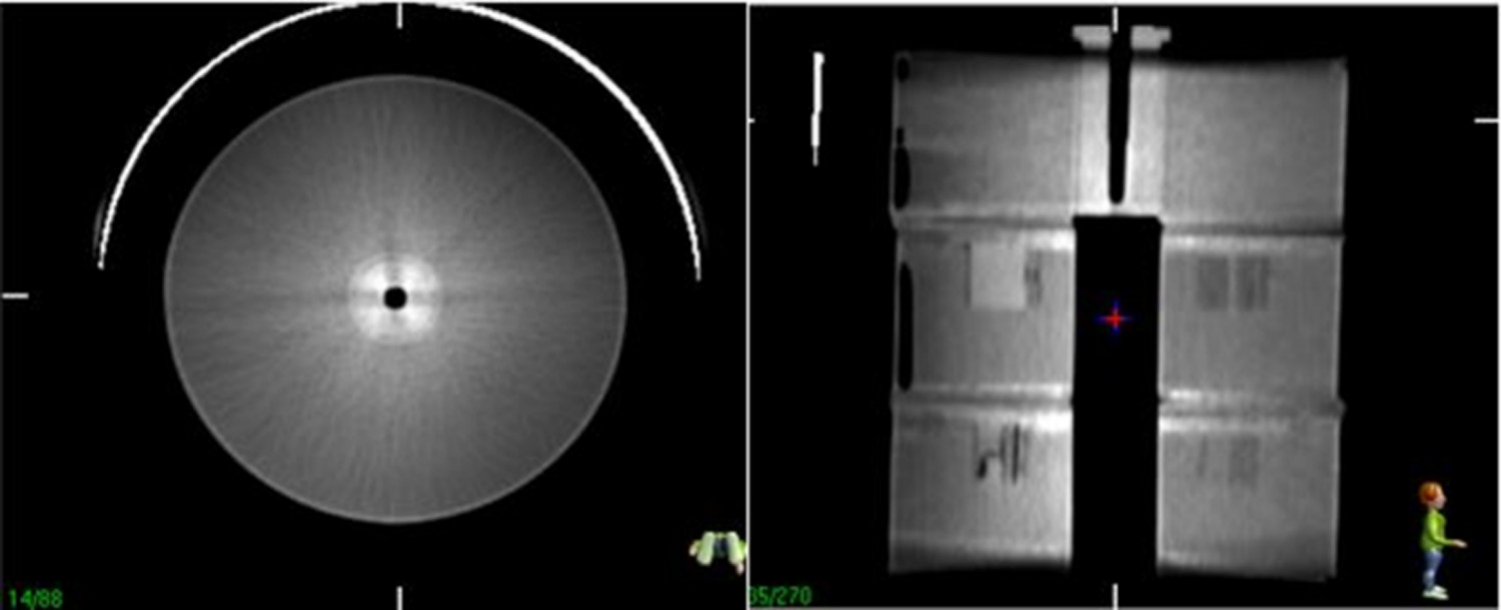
Cone-beam computed tomography (CBCT) images of the water-filled uniformity slab.
